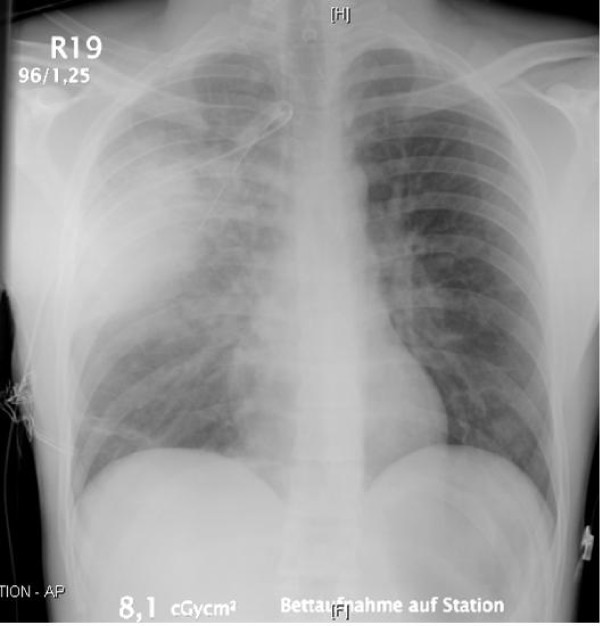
The second chest x-ray with the thoracic drain. The lung is correctly expanded. There is a suspect shadow along the lateral right chest wall.
